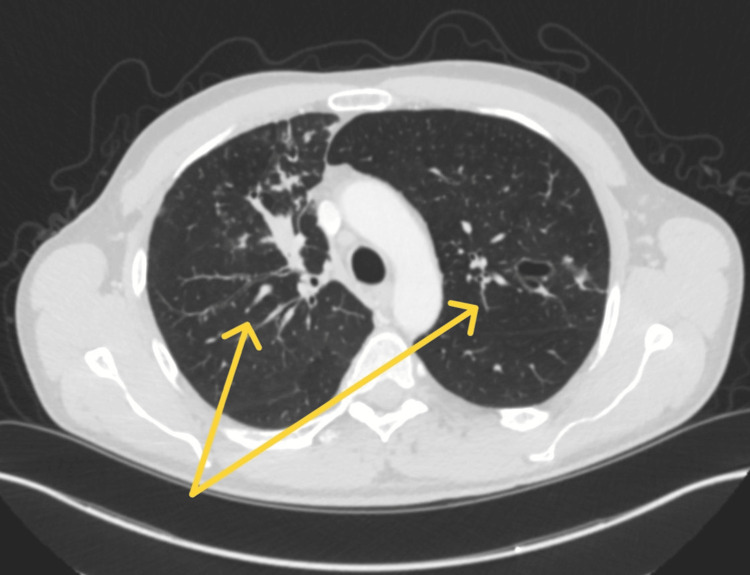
Post-discharge CT Chest With Contrast Yellow arrows: bronchiectasis with associated nodular branching and tree-in-bud opacities, as well as lobular soft tissue nodules in the left upper lobe.
